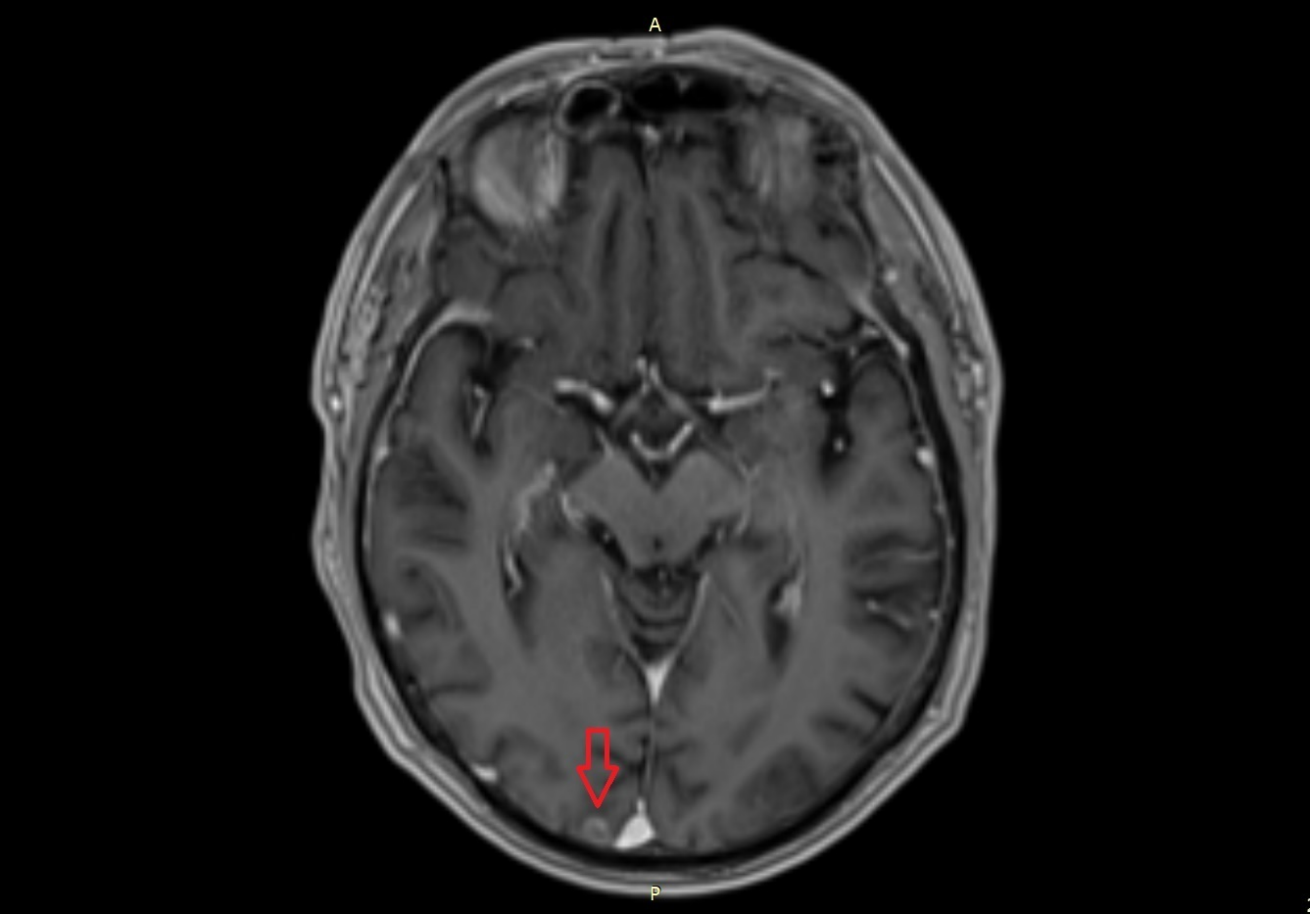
MRI scan. The image shows the suspect lesion in the right occipital lobe. MRI: magnetic resonance imaging
